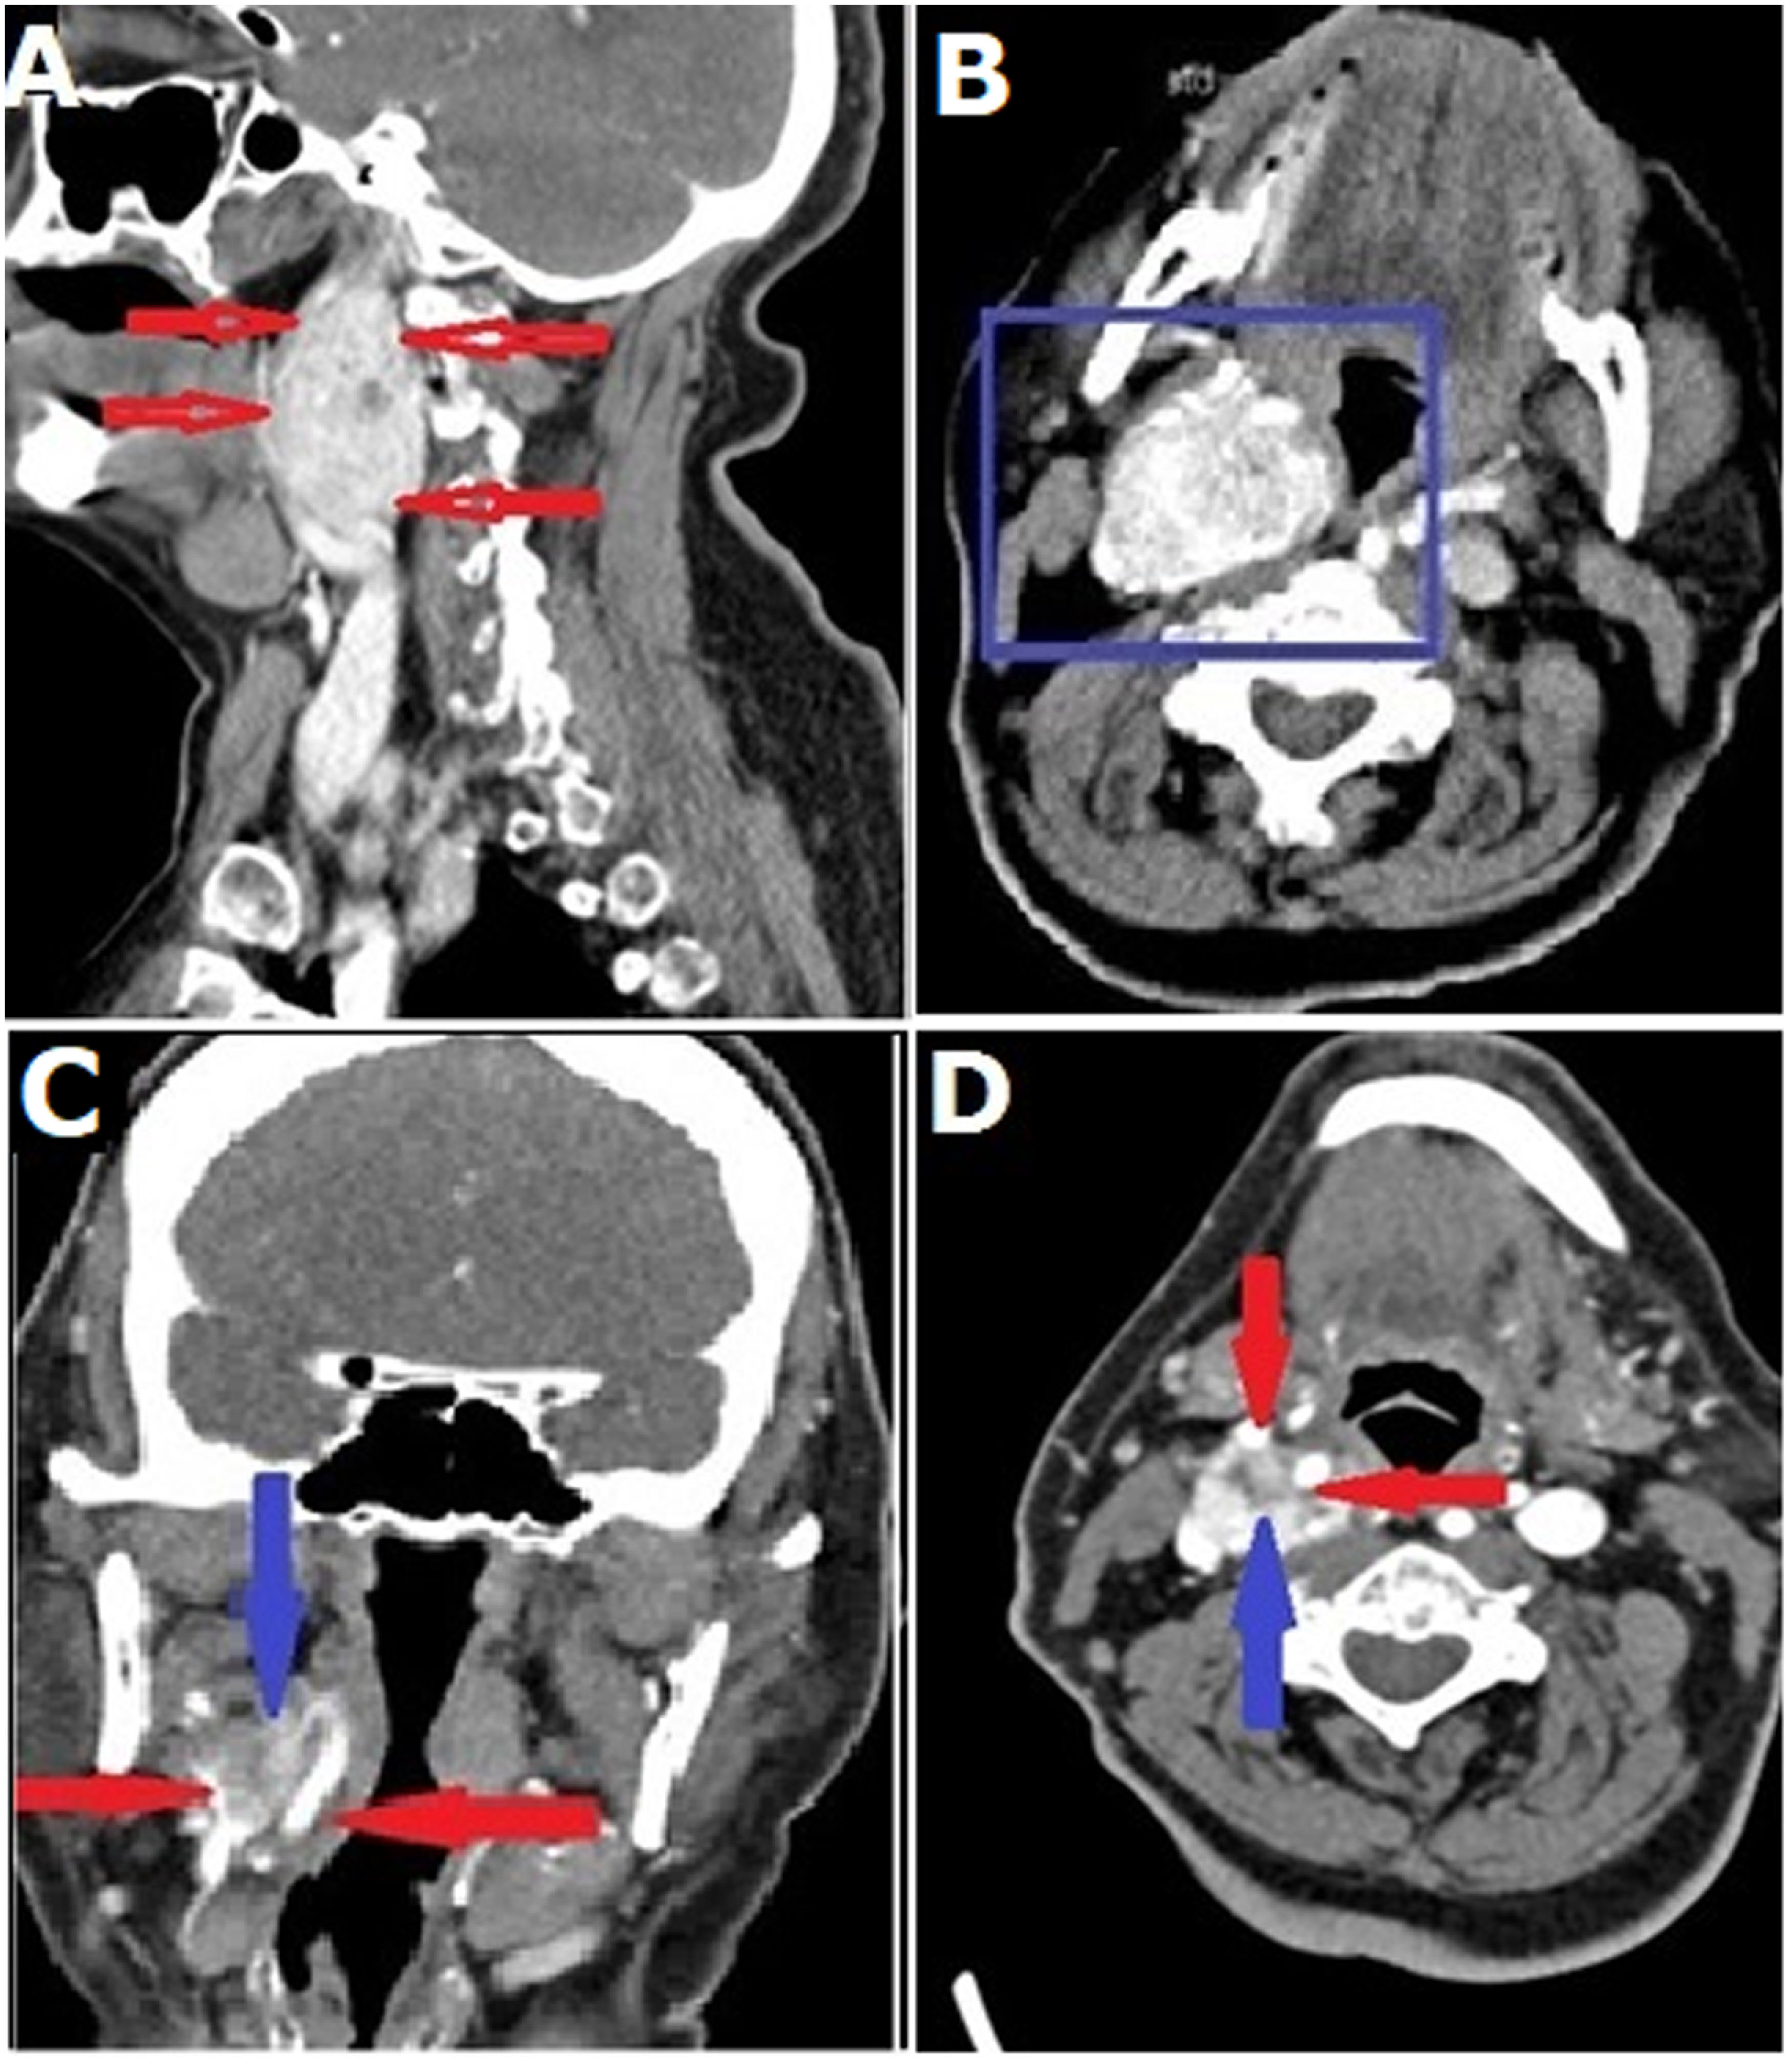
Fig. 1 Contrast-enhanced computed tomography (CT) with early arterial phase contrast shows a heterogeneously enhancing mass at the right carotid bifurcation. (A) The sagittal section depicts a giant carotid body tumor causing bending of the branches of the carotid artery (red arrows). (B) In the axial section of CT angiography, there is an image of the internal and external carotid arteries pushed due to the presence of the tumor (blue frame). (C) In the coronal section of the neck CT angiography taken 2 days after embolization, it can be seen that the intense contrast retention of the tumor formation decreases, the mass is reduced, and the internal-external carotid artery branches are clearly prominent (red and blue arrows). (D) It was found that intense contrast enhancement decreased in the tumor formation image, the mass became smaller, and the internal-external carotid artery branches were clearly seen in the axial sections (red and blue arrows).
